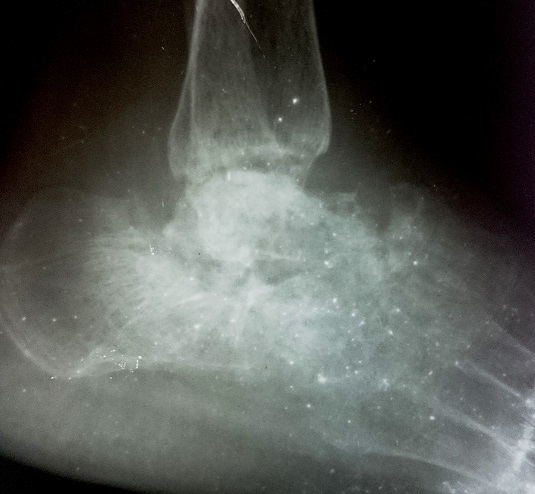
Radiographie de la cheville de face (à 06 mois) image séquellaire, ankylose
